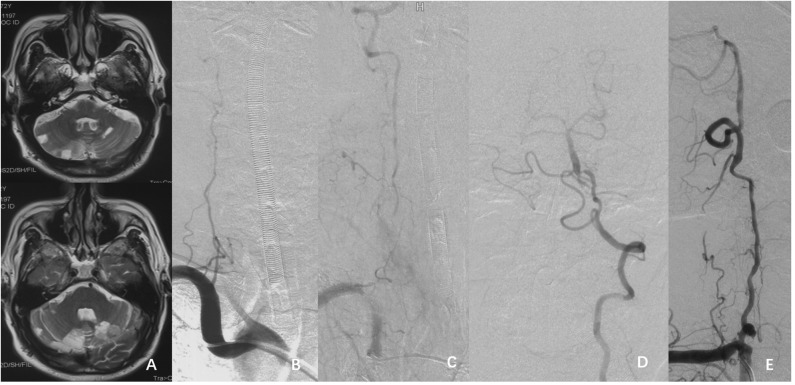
(A) MRI T2 weighted image showing multiple infarctions in the bilateral cerebellar hemispheres. (B–D) DSA showing the right VA occlusion and the dysplasia in the left V4 segment. (E) Follow-up DSA showed the patency of right VA 1-year postoperatively.
